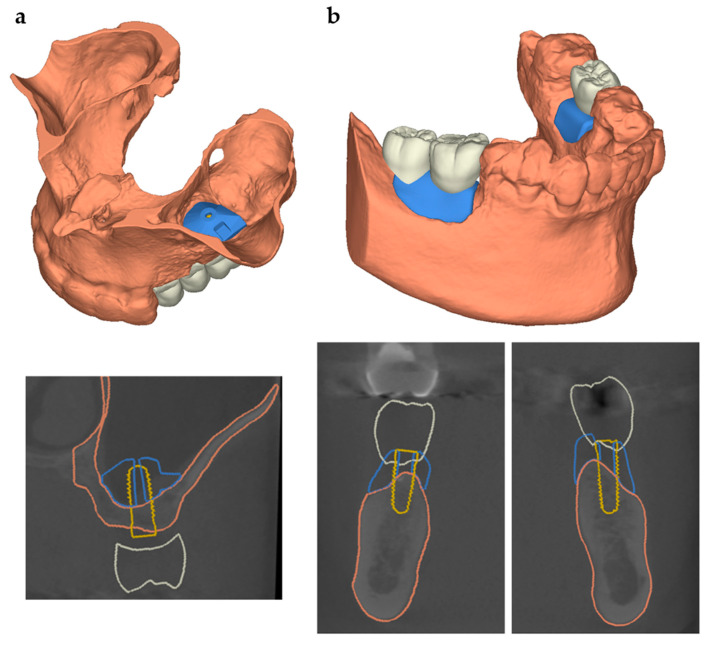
Digital backward planning based on CBCT data. (a) Sinus floor elevation in region 26, (b) bilateral onlay graft in the posterior mandible (white: prosthetic restoration; yellow: dental implant; orange: bone contour; blue: planned CPC scaffold.
